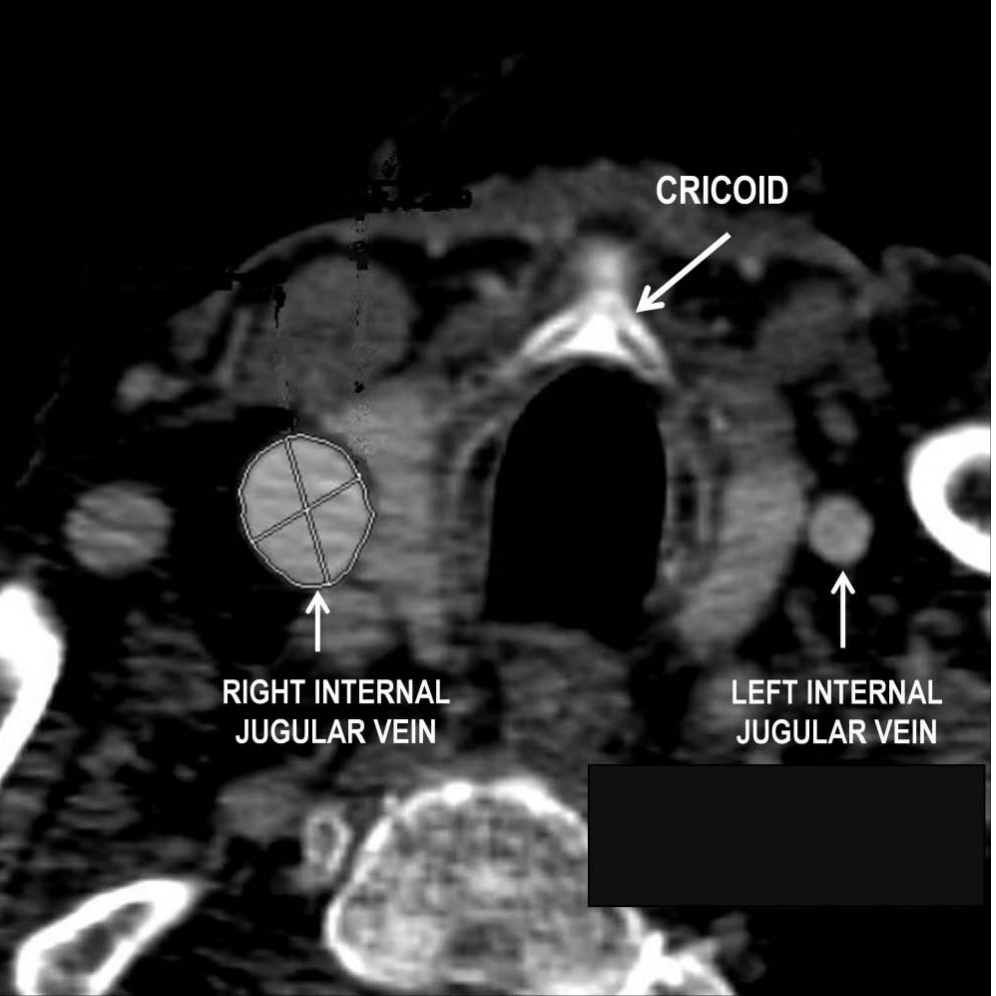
Example of a thoracic contrast-enhanced helical computed tomography scan obtained from a patient with a large right internal jugular vein.
